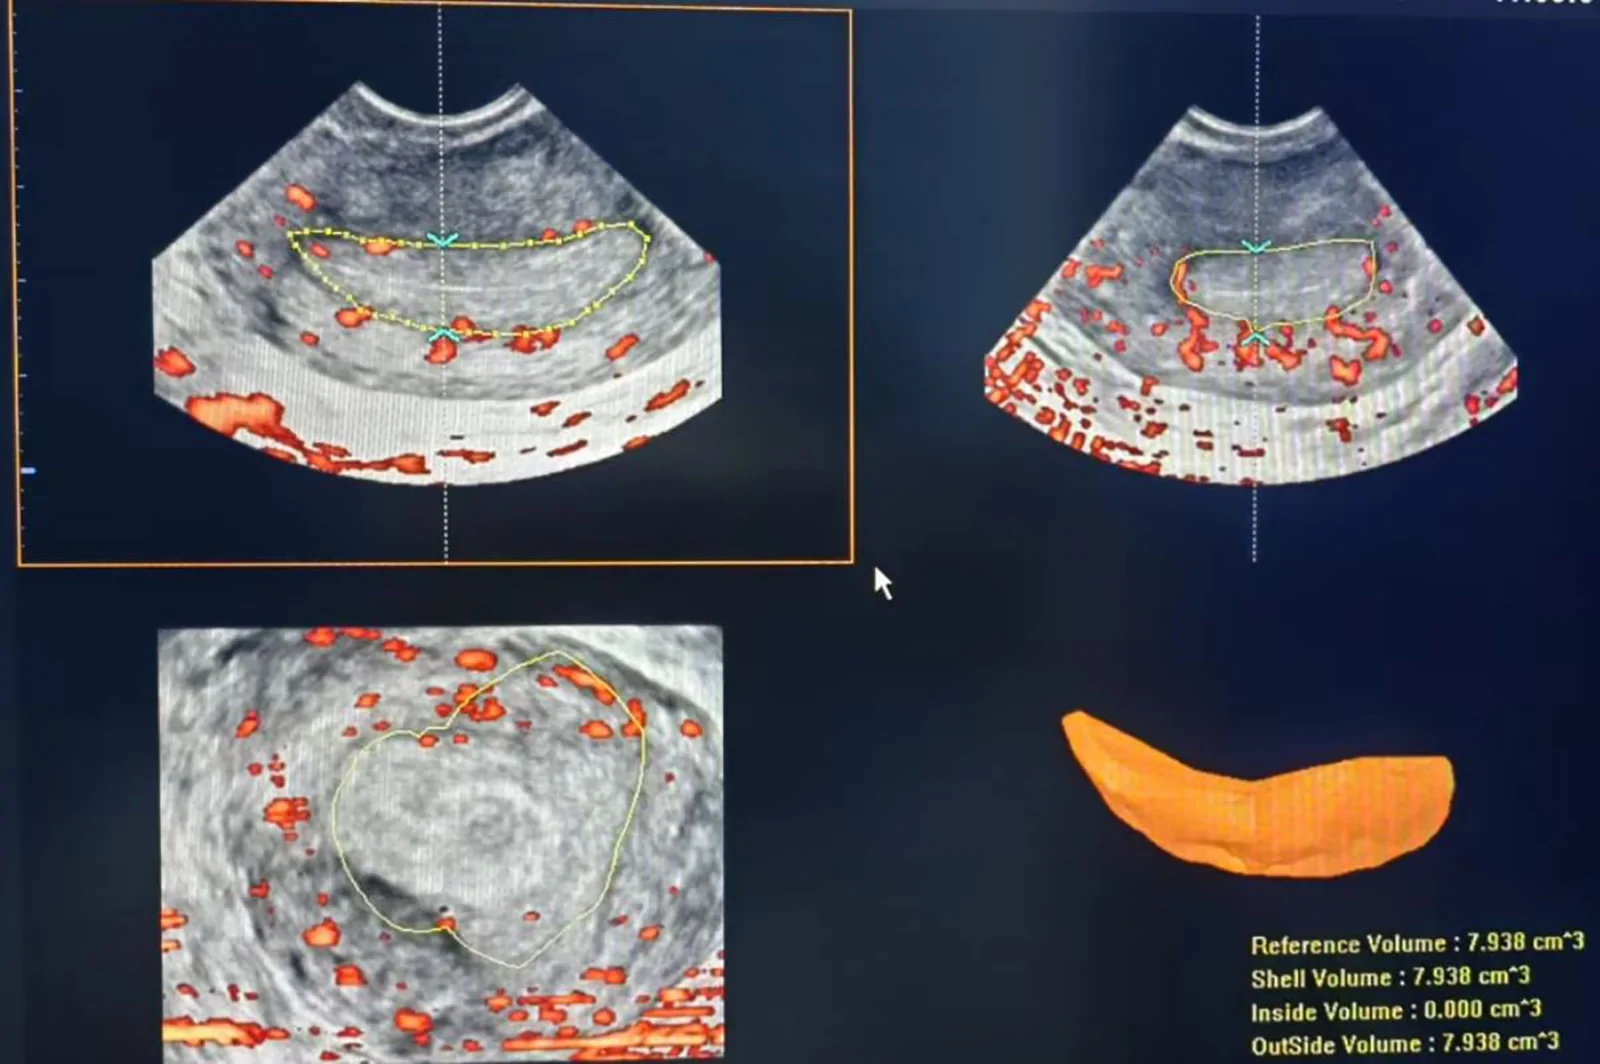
Measurement of endometrial volume in patients with ADM using 3D ultrasound. 3D = three-dimensional, ADM = adenomyosis.
